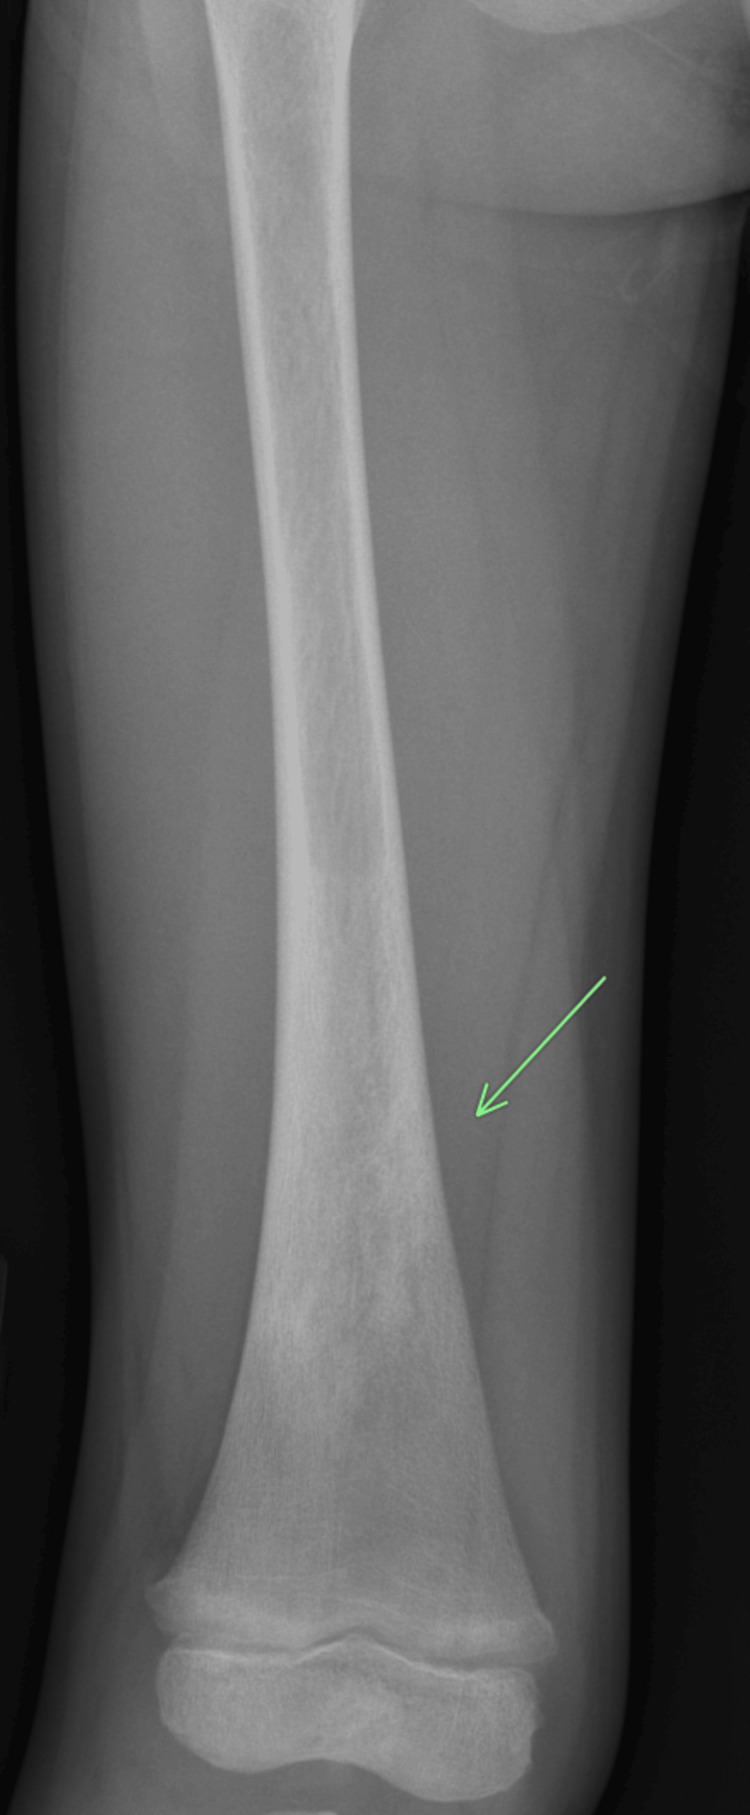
Anteroposterior X-ray distal right femur Seen is a heterogeneous lytic and sclerotic region in the distal diaphysis (oblique arrow).
